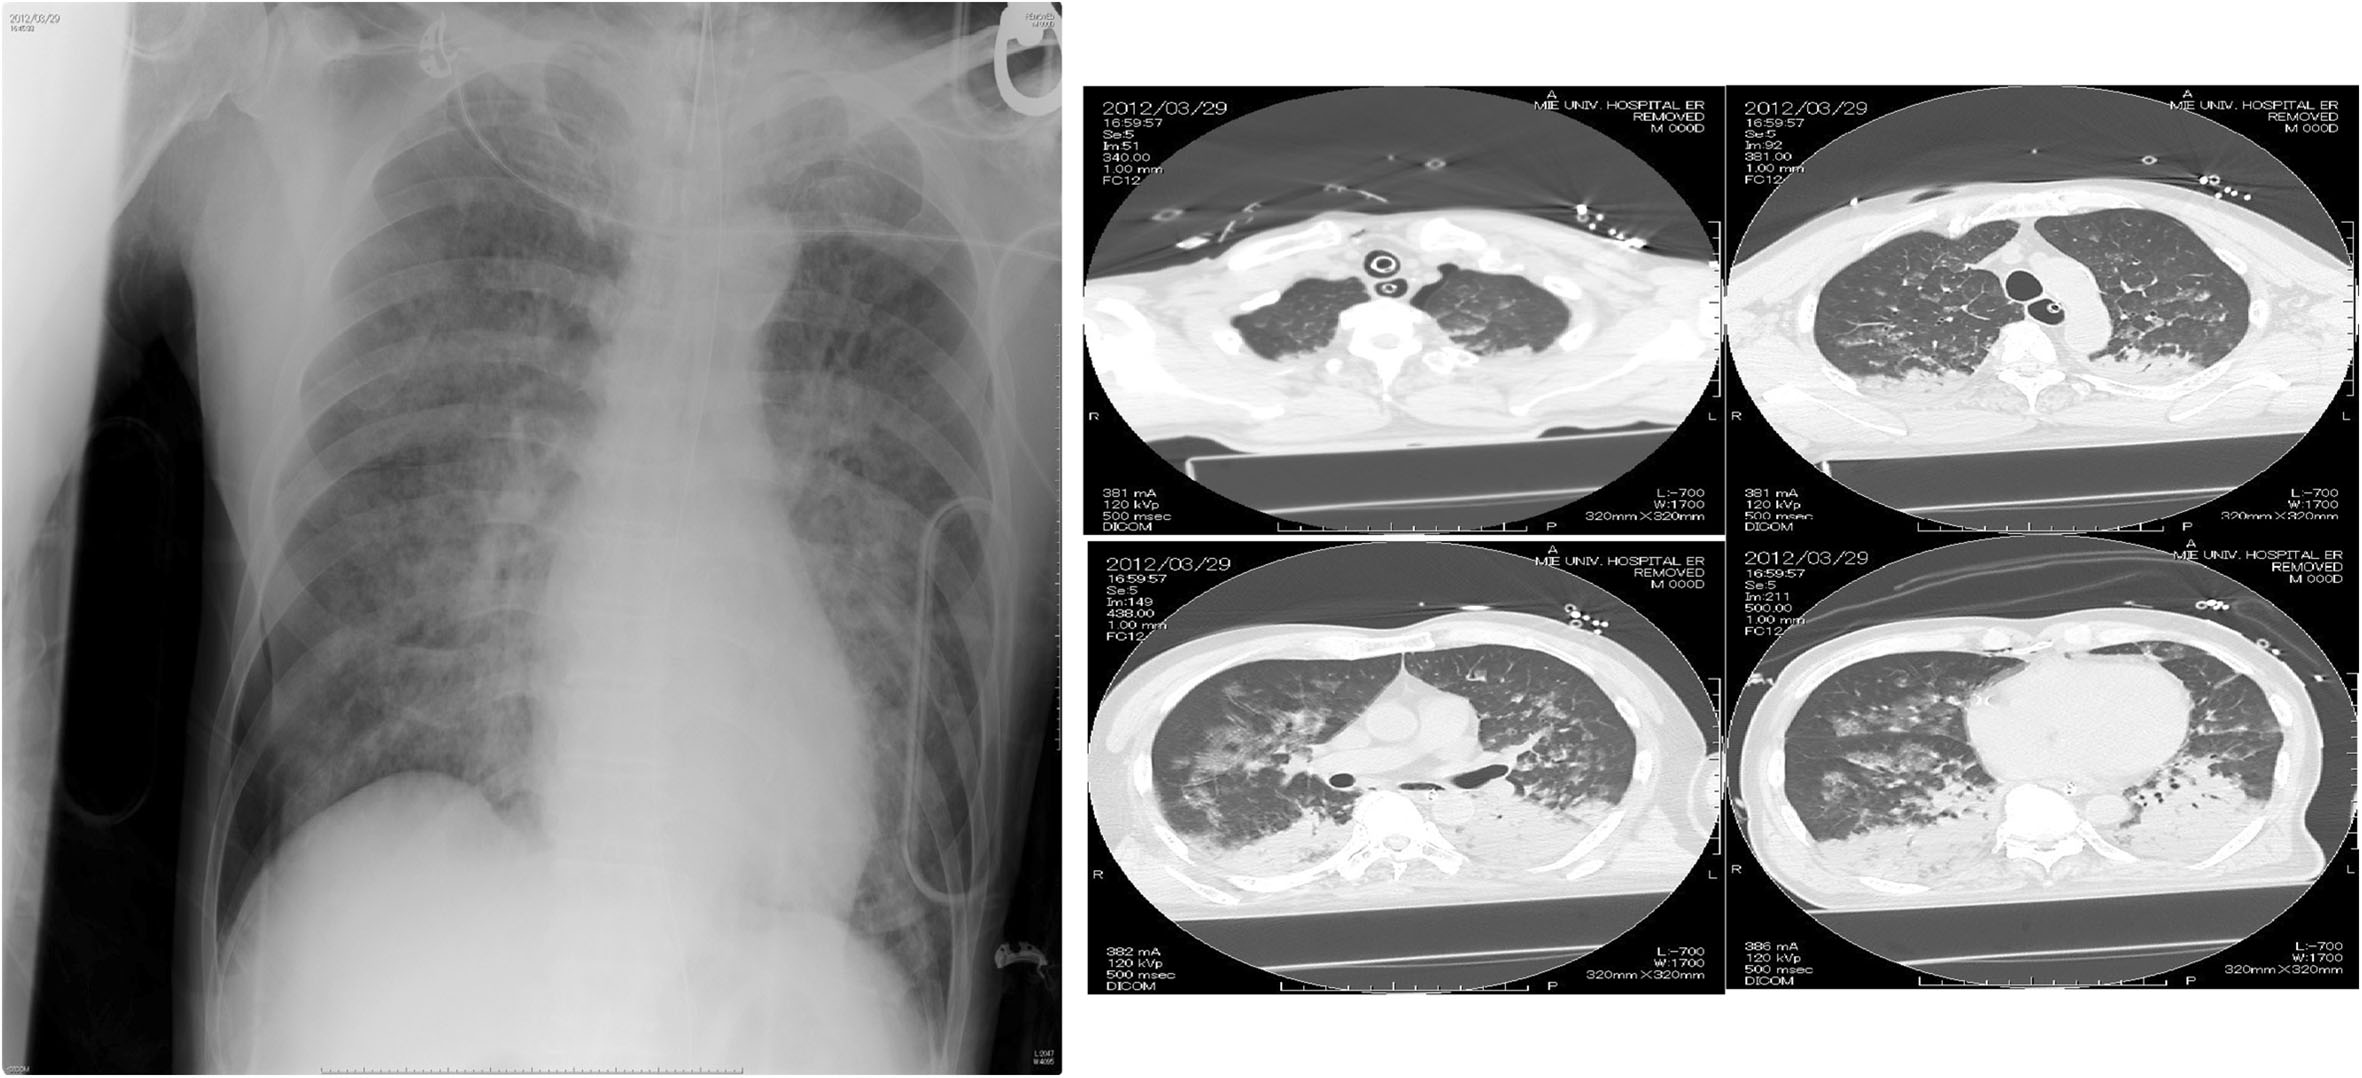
Chest X-ray and CT scan upon ICU admission. Chest radiograph shows diffuse infiltrate bilaterally.
